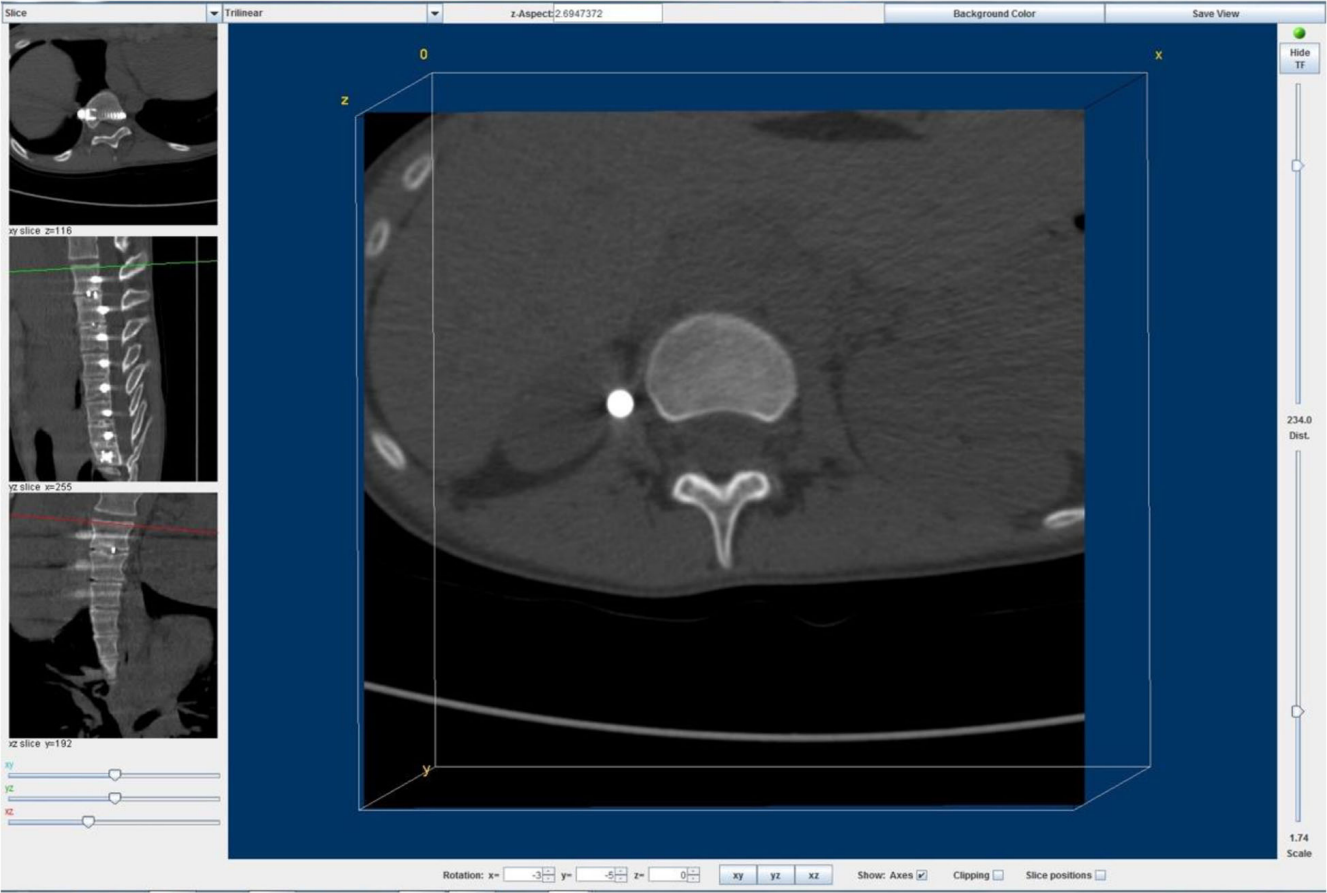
An example of a true axial image of the inferior endplate of L1. The large, true axial, image is obtained by modifying the plane of viewing in the frontal and sagittal planes with respect to the smaller images to the left. Rotation in the transverse plane was analysed in relation to the arbitrary CT scanner bed plane, thus allowing the measurement of relative inter-endplate rotations within patients
